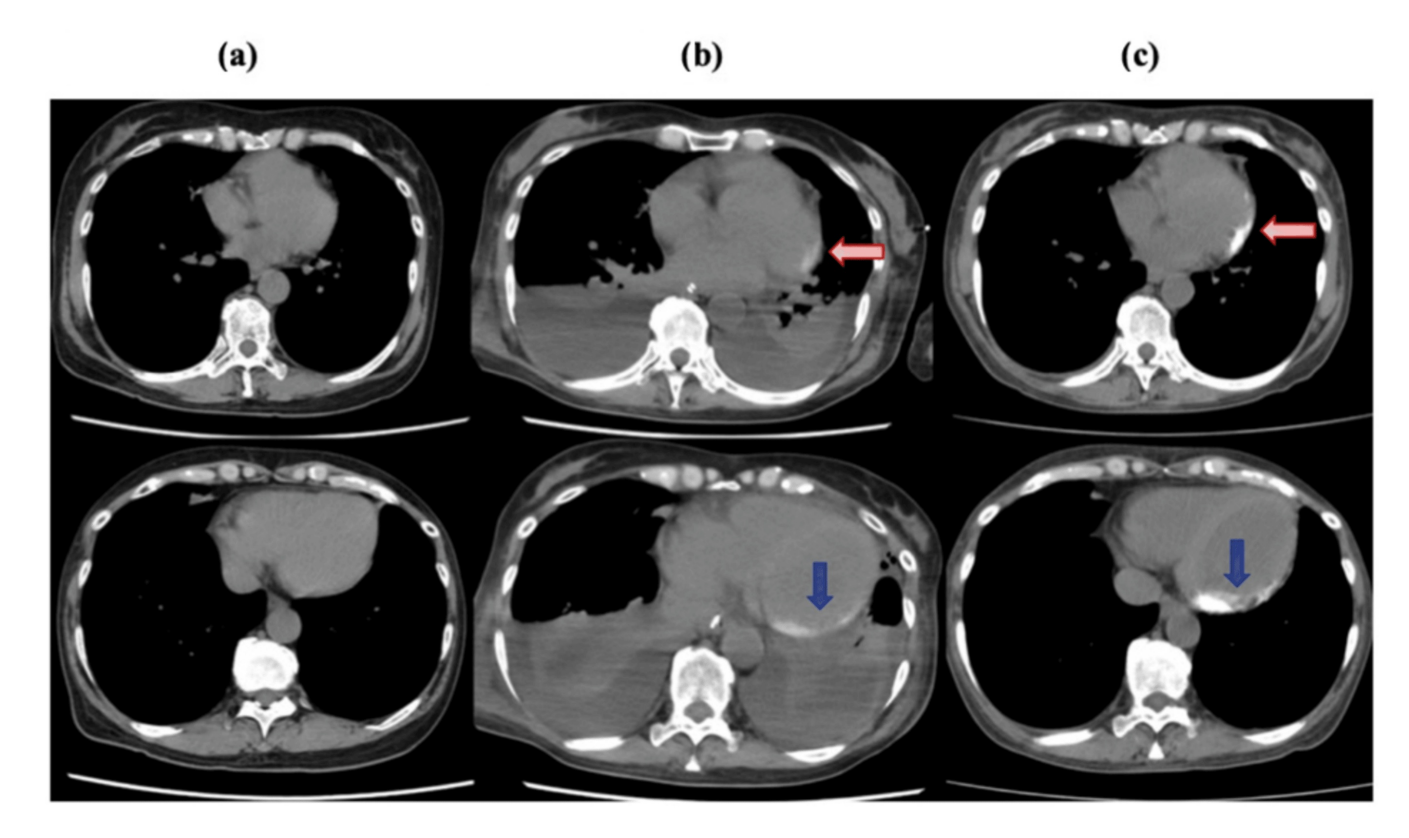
Newly developed high-density area on the pericardium observed on CT. (a) Thirty-five days prior to CHOP therapy: No abnormal high-density area observed. (b) One week after CHOP therapy: A newly developed high-density area appeared on the pericardium along the lateral (red arrow) and inferior walls (blue arrow) of the heart. (c) Two months after CHOP therapy: The previously noted high-density areas on the lateral (red arrow) and inferior (blue arrow) pericardium became more prominent, raising suspicion of pericardial calcification. CT: computed tomography.
